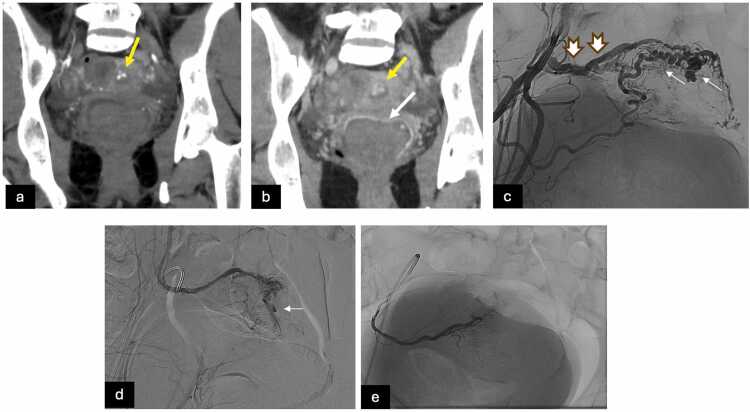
Status post stillbirth at 30 weeks. Complex recovery including retained products of conception 6 months post birth presented with heavy vaginal bleeding a) Coronal CT Angiogram showing pseudoaneurysm in the cavity and clot in the vaginal vault (yellow Arrow) b) Portovenous Phase showing active bleed into cavity (yellow arrow) and contrast extending around the existing clot in the vault (white arrow) c) Angiogram of the right uterine artery (white arrows) shows venous fistula formation with uterine vein (arrow heads) d) Late phase of the angiogram also shows active bleeding (white arrow) from the left side of the uterine artery e) Particle embolization performed using PVA (polyvinyl alcohol). Diagnosis: Pseuodaneurysm and venous fistula.
